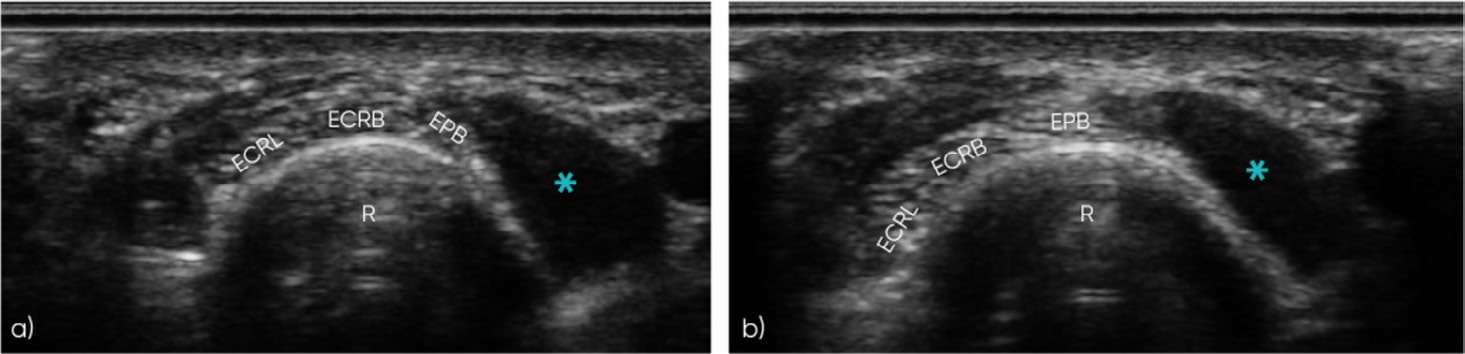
Dynamic ultrasound assessment of the extensor pollicis brevis muscle at the proximal intersection. (a, b) Short‐axis ultrasound images of the symptomatic limb. (a) At rest, the second compartment tendons (ECRL and ECRB) remain almost centrally aligned. (b) Contraction of the EPB muscle, during extension of the metacarpophalangeal joint, results in significant radial displacement of the second compartment tendons. *R*, radius; ECRL, extensor carpi radialis longus tendon; ECRB, extensor carpi radialis brevis tendon; EPB, extensor pollicis brevis tendon; blue asterisk, extensor pollicis brevis muscle.
